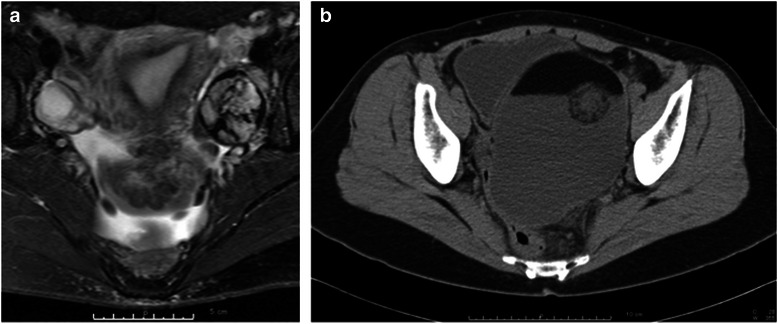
Image analysis. a Gadolinium-enhanced T1-weighted magnetic resonance imaging scan of the left ovary shows a cystic lesion, approximately three-fourths of which is occupied by an irregularly enhanced mass. b An enhanced computed tomography scan shows a large intra-abdominal cystic lesion occupying the pelvic cavity. Focal intracystic irregular enhancement is noted
